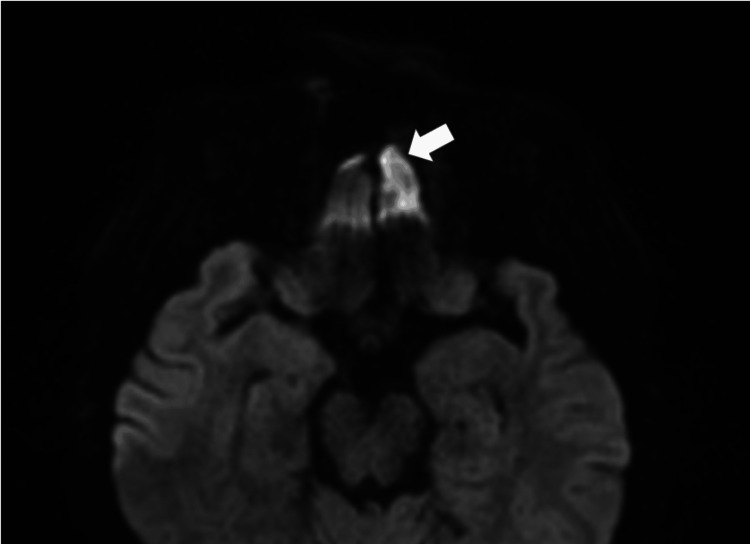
MRI diffusion restriction in the left gyrus rectus suggestive of inflammation/intracranial fungal invasion/or septic embolism MRI: magnetic resonance imaging
